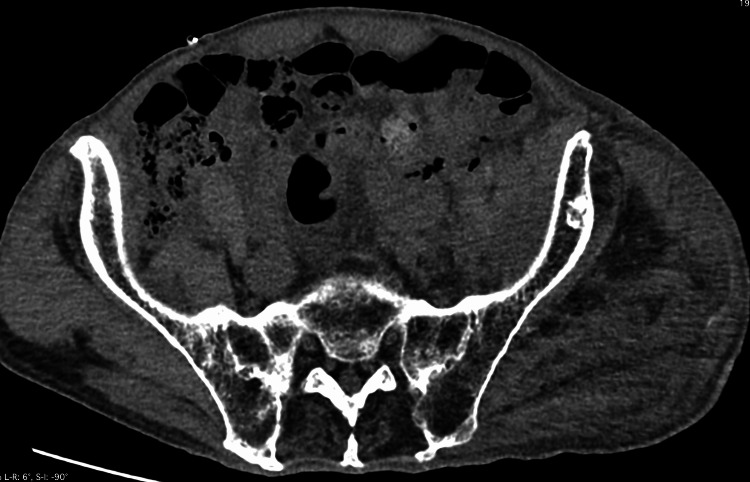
Computed tomography image The day after the first operation computed tomography revealed no intrapelvic bleeding
